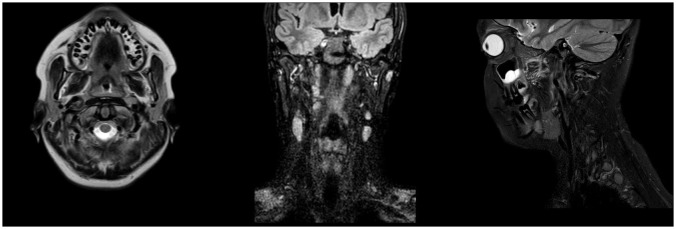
Post-operative Magnetic Resonance Imaging (MRI). Axial T2-weighted (left), coronal FLAIR (middle), and sagittal T2-weighted (right) post-operative MRI scans demonstrating complete excision of the hypoglossal schwannoma at 6-month follow-up.
